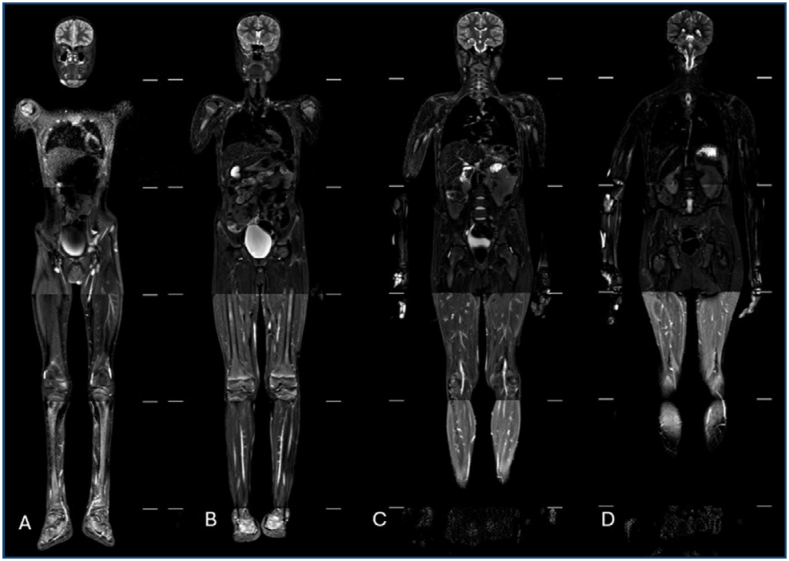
(A–D) 12-year-old boy with synovial sarcoma: consecutive coronal whole-body magnetic resonance imaging short tau inversion recovery images (A–D) through whole body show no metastasis or recurrence on follow-up.
